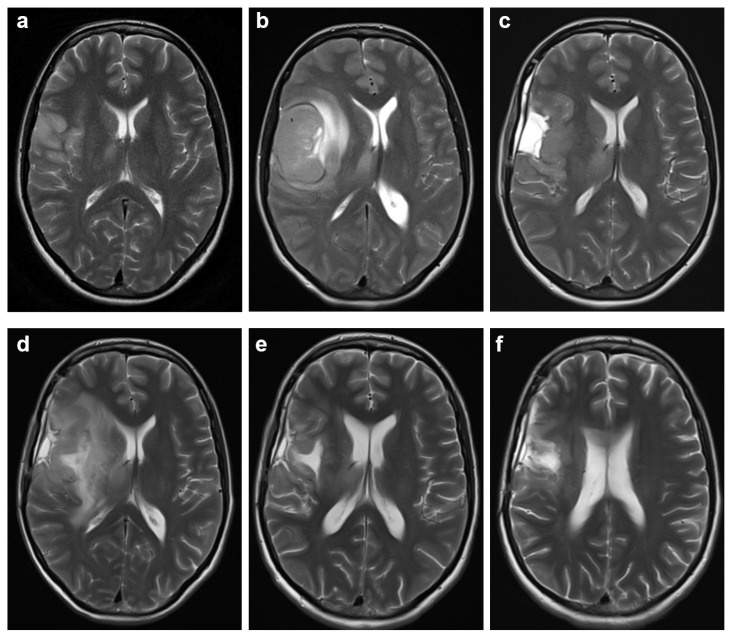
Sequential T2-weighted axial brain MRIs over a 12-month period, corresponding to the timeline shown in Figure 1. (a) MRI at 24 weeks of pregnancy showing a hyperintense lesion in the right frontal lobe. (b) MRI at 33 weeks of pregnancy showing significant enlargement of the lesion. (c) Postoperative MRI five weeks after tumor resection. (d) MRI four months after resection showing tumor recurrence and progression despite surgery and radio-chemotherapy (RT + TMZ). (e) MRI seven months after resection, following two cycles of lomustine and four administrations of bevacizumab, demonstrating radiological regression. (f) MRI nine months after resection showing further tumor regression following three cycles of lomustine and nine administrations of bevacizumab, along with six doses of personalized anticancer vaccine administered abroad.
